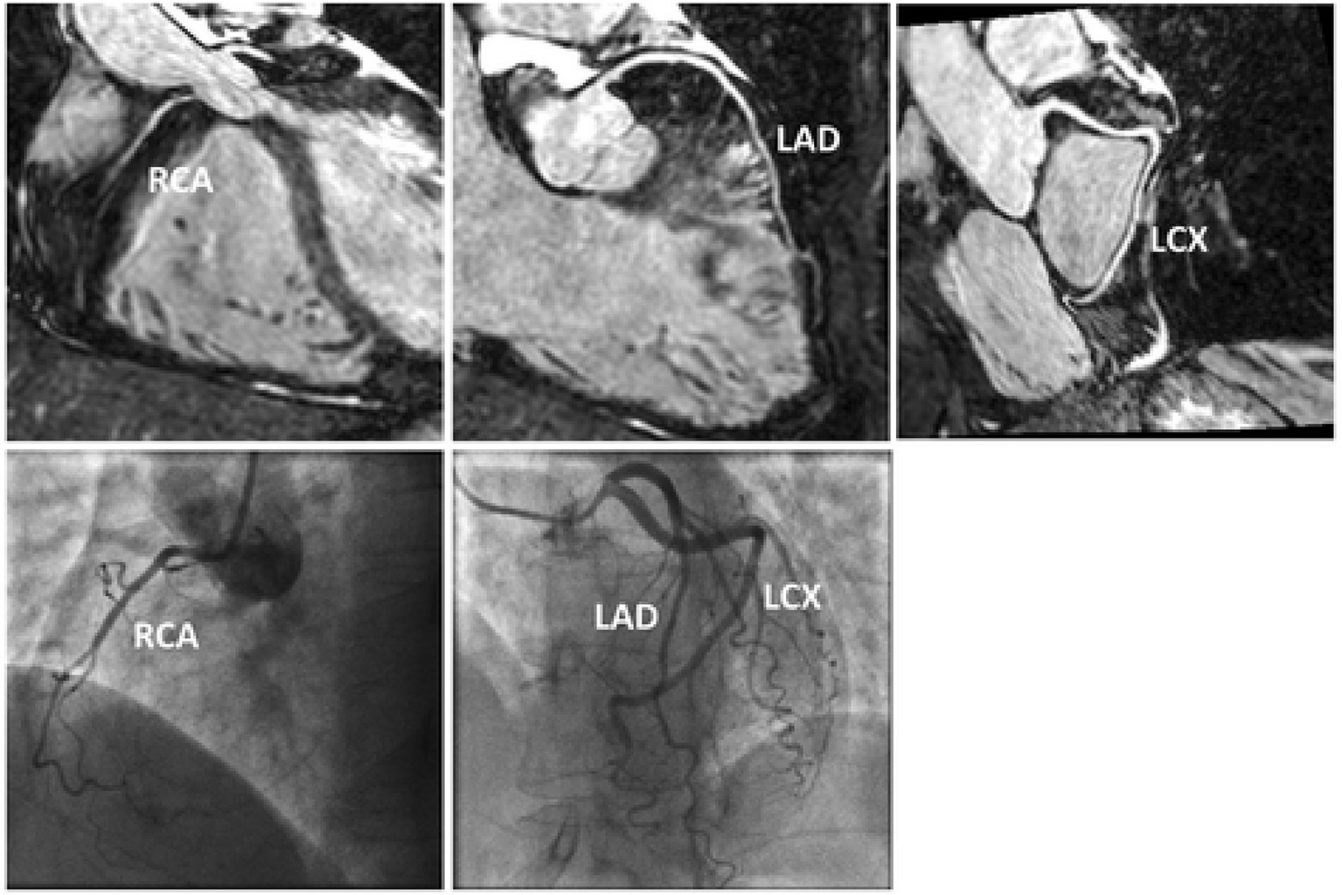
Reformatted CMRA datasets (top row) from a patient without coronary artery disease but non dominant RCA. Coronary x-ray angiography in the same patient (bottom row). RCA, right coronary artery; LAD, left anterior descending artery; LCX, left circumflex artery. Adapted with permission from Henningsson et al. (52).
